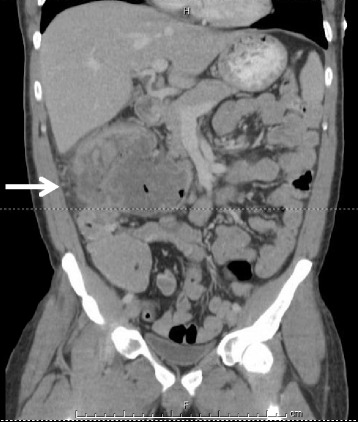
Computed tomography with intravenous and oral contrast from case 2. Coronal section of the abdomen and pelvis. *Arrow* points to 14.0 × 9.8 × 10.8 cm heterogeneous mass in the right upper quadrant abutting the ascending colon and duodenum. Oral contrast is seen within the mass. The fat plane between the mass and the second segment of the duodenum is obscured
